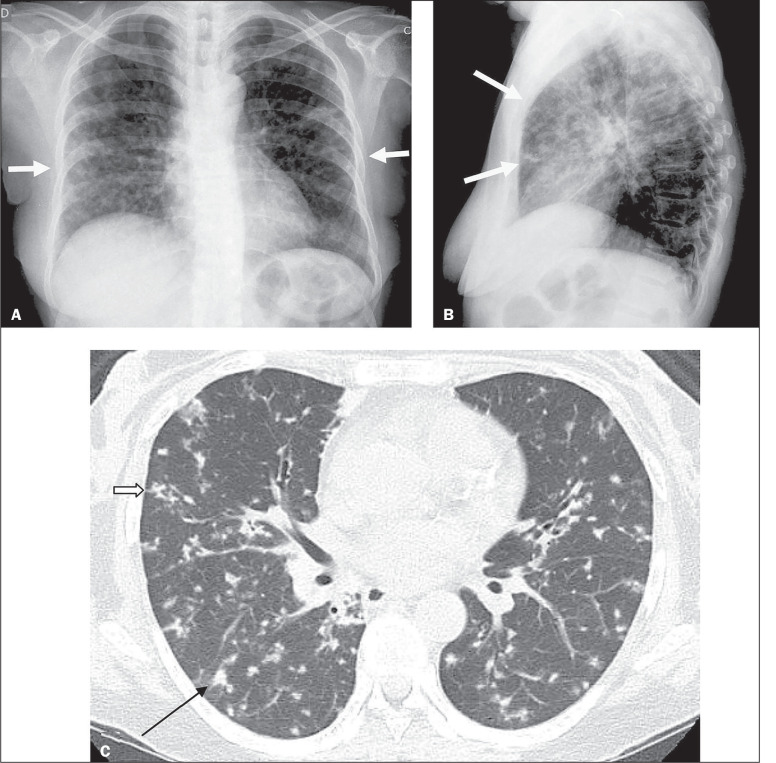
Chest X-ray (A,B) and HRCT (C) of a 41-year-old female with pulmonary TB (patient
